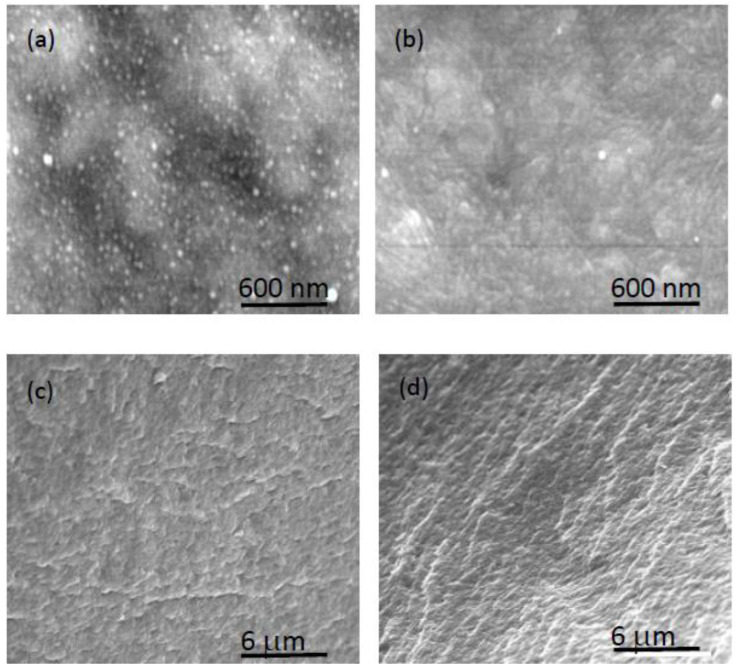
SEM images from a pristine 50%AlqNO_3_–50%CTA PIM: surface (a) and cross-section (c). The same membrane after immersion in ultrapure water: surface (b) and cross-section (d).
